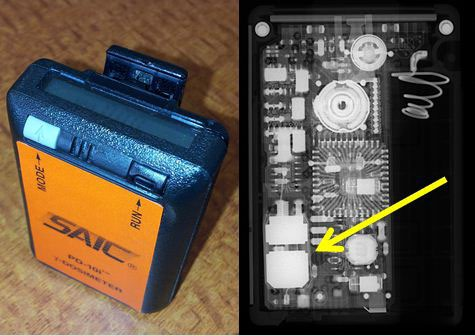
PD‐10i EPD (left) and radiograph (right). Arrow indicates the GM tube.
